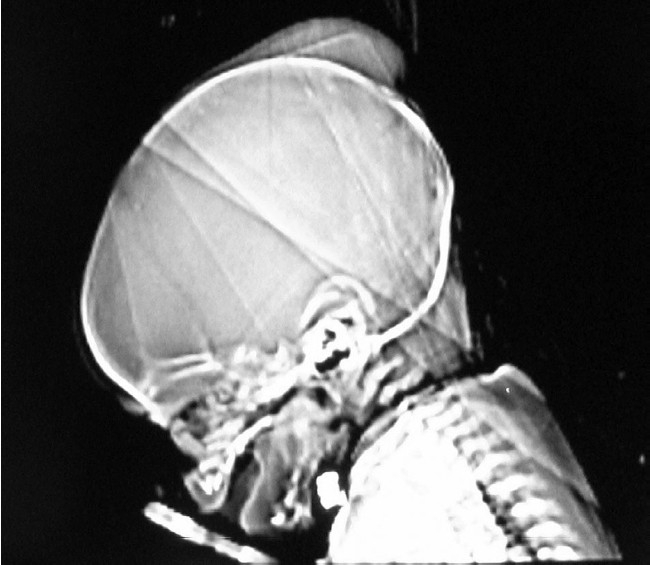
Plain radiograph demonstrating well-circumscribed smooth mass overlying the skull, consistent with sub-aponeurotic CSF collection. There were no signs of skull fracture, abnormal areas of lucency or sclerosis
